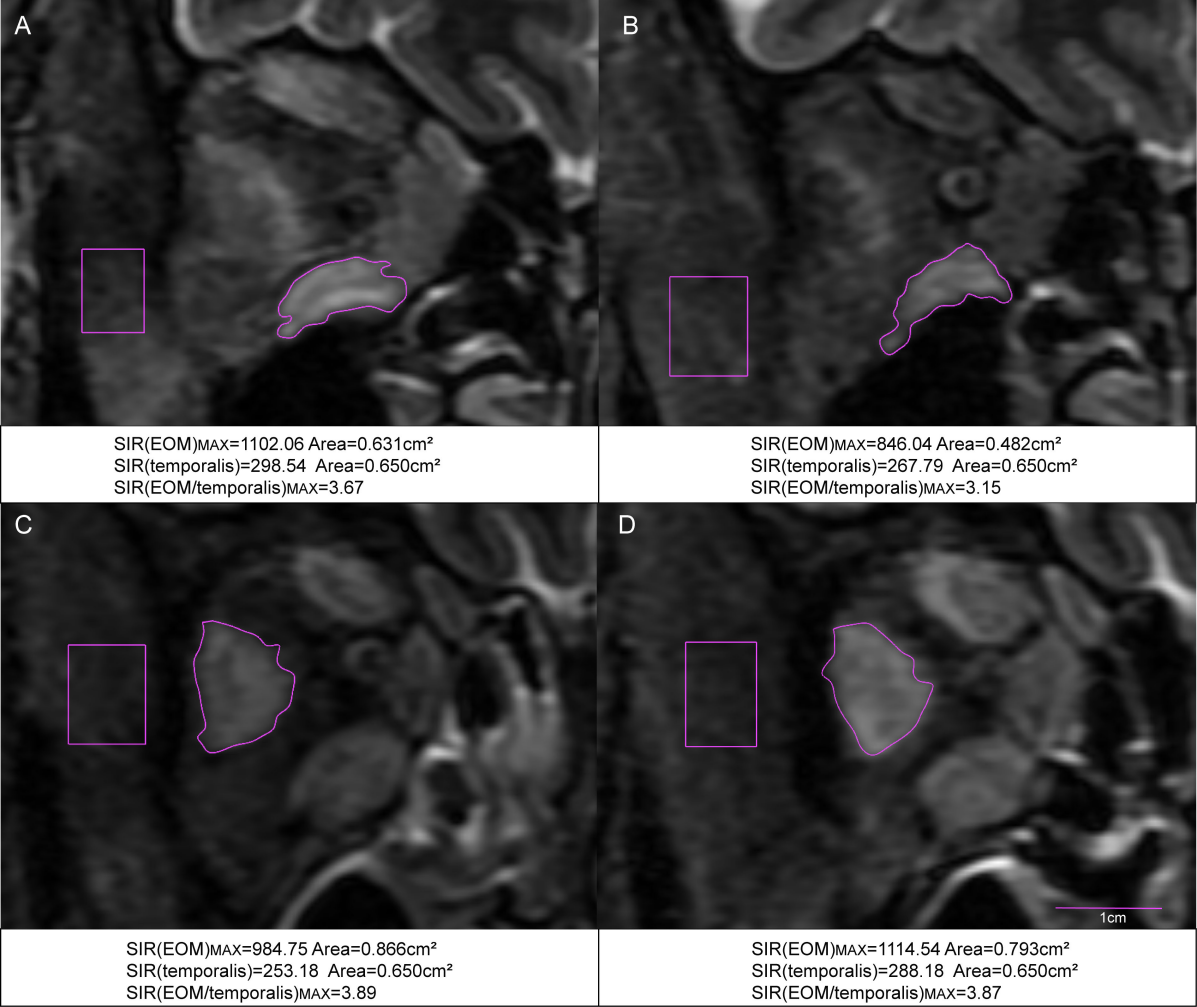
Coronal STIR MRI scans of the extraocular muscles in TAO patients with hormone-resistant DON. (A) Pre-treatment MRI of the right eye in a patient who did not require surgery. (B) Post-treatment MRI of the same patient after one course of TCZ treatment. (C) Pre-treatment MRI of the right eye in a patient who underwent surgery. (D) Post-treatment MRI of the same patient after one course of TCZ treatment. SIR(EOM/temporalis)_MAX_: the maximal signal intensity ratio of extraocular muscle to temporalis muscle.
